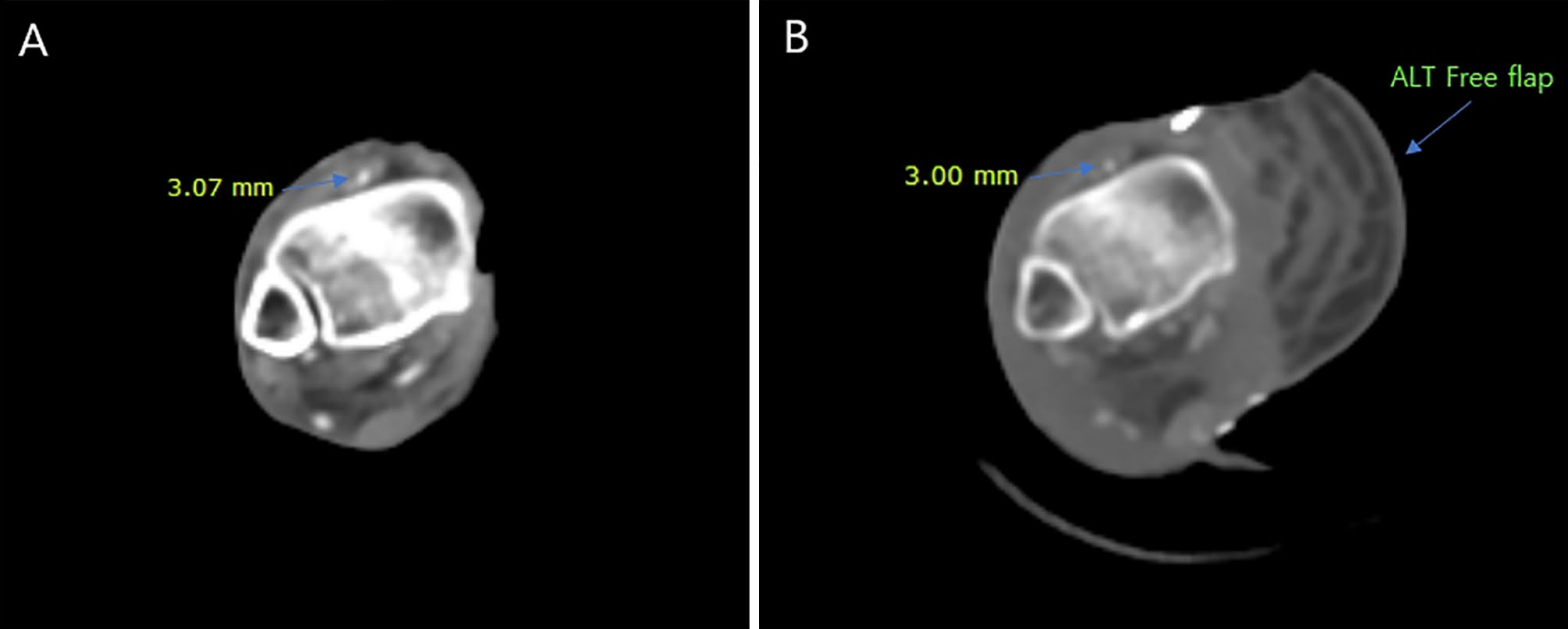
Computed Tomography (CT) angiography finding. Patent flap pedicle was confirmed without the evidence of compression. The diameter of each pedicle was measured. (A) Before NPWT system apply, DD* = 3.07 mm (B) After NPWT system apply, DD = 3.00 mm. DD* = Diameter of Dorsalis pedis artery
